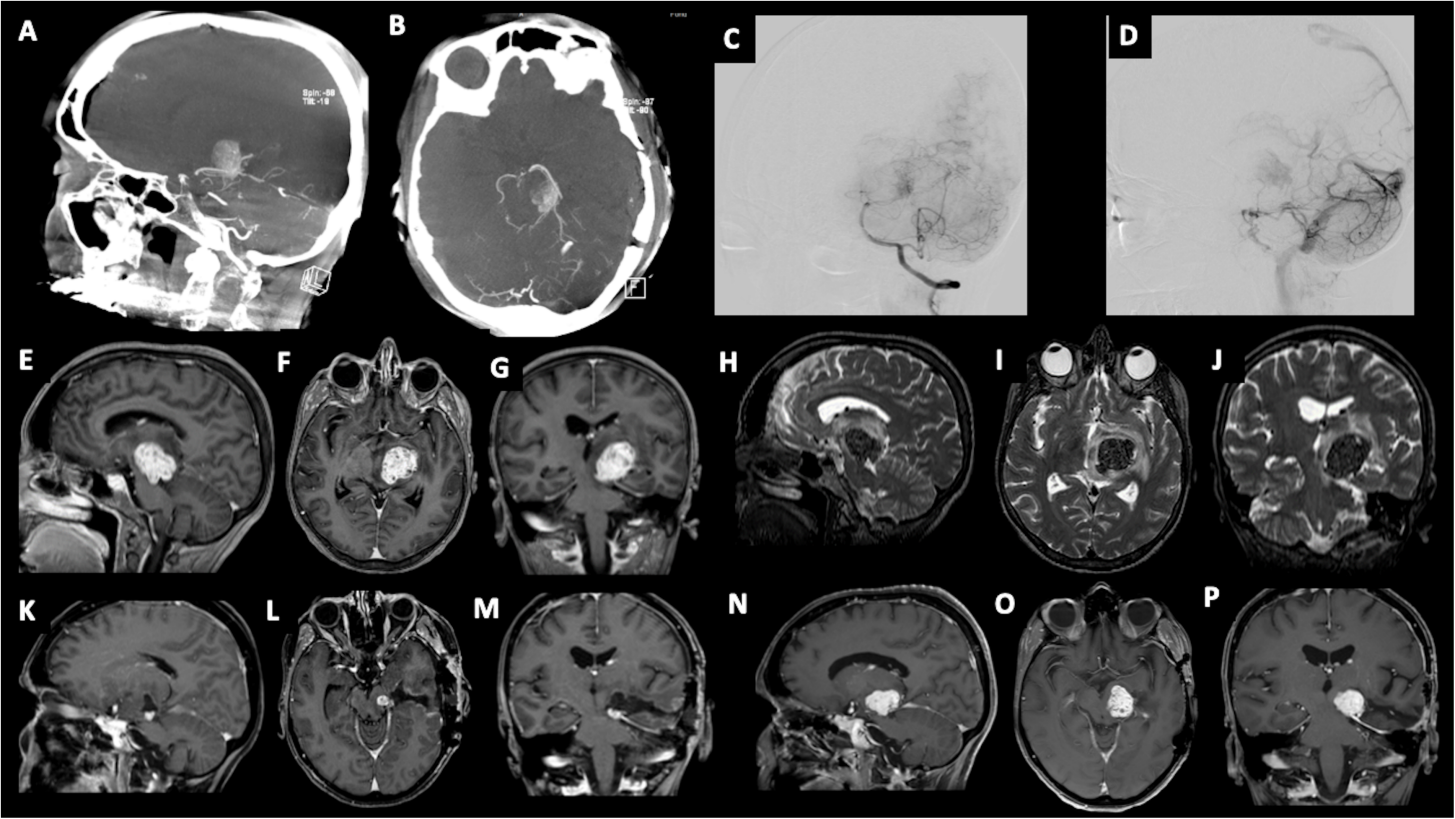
(A–D) Angiography imaging showing artero-venous blush lasting till late venous phase. The blush is fed by collaterals originating from P2 segment of the posterior cerebral artery (PCA), and left superior cerebellar artery (SCA). Preoperative post-contrast T1-weighted images in sagittal (E), axial (F), and coronal (G) views, showing the involvement of the tentorium hiatus and the extension to the left thalamo-mesencephalic region; Preoperative T2-weighted images in sagittal (H), axial (I), and coronal (J) views, showing the hypointensity of the tumor and the presence of perilesional edema. Post-operative post-contrast T1-weighted imaging showing residual tumor in sagittal (K), axial (L), and coronal views (M); (N-P) 5-month follow-up post-contrast T1-weighted imaging showing disease recurrence.
